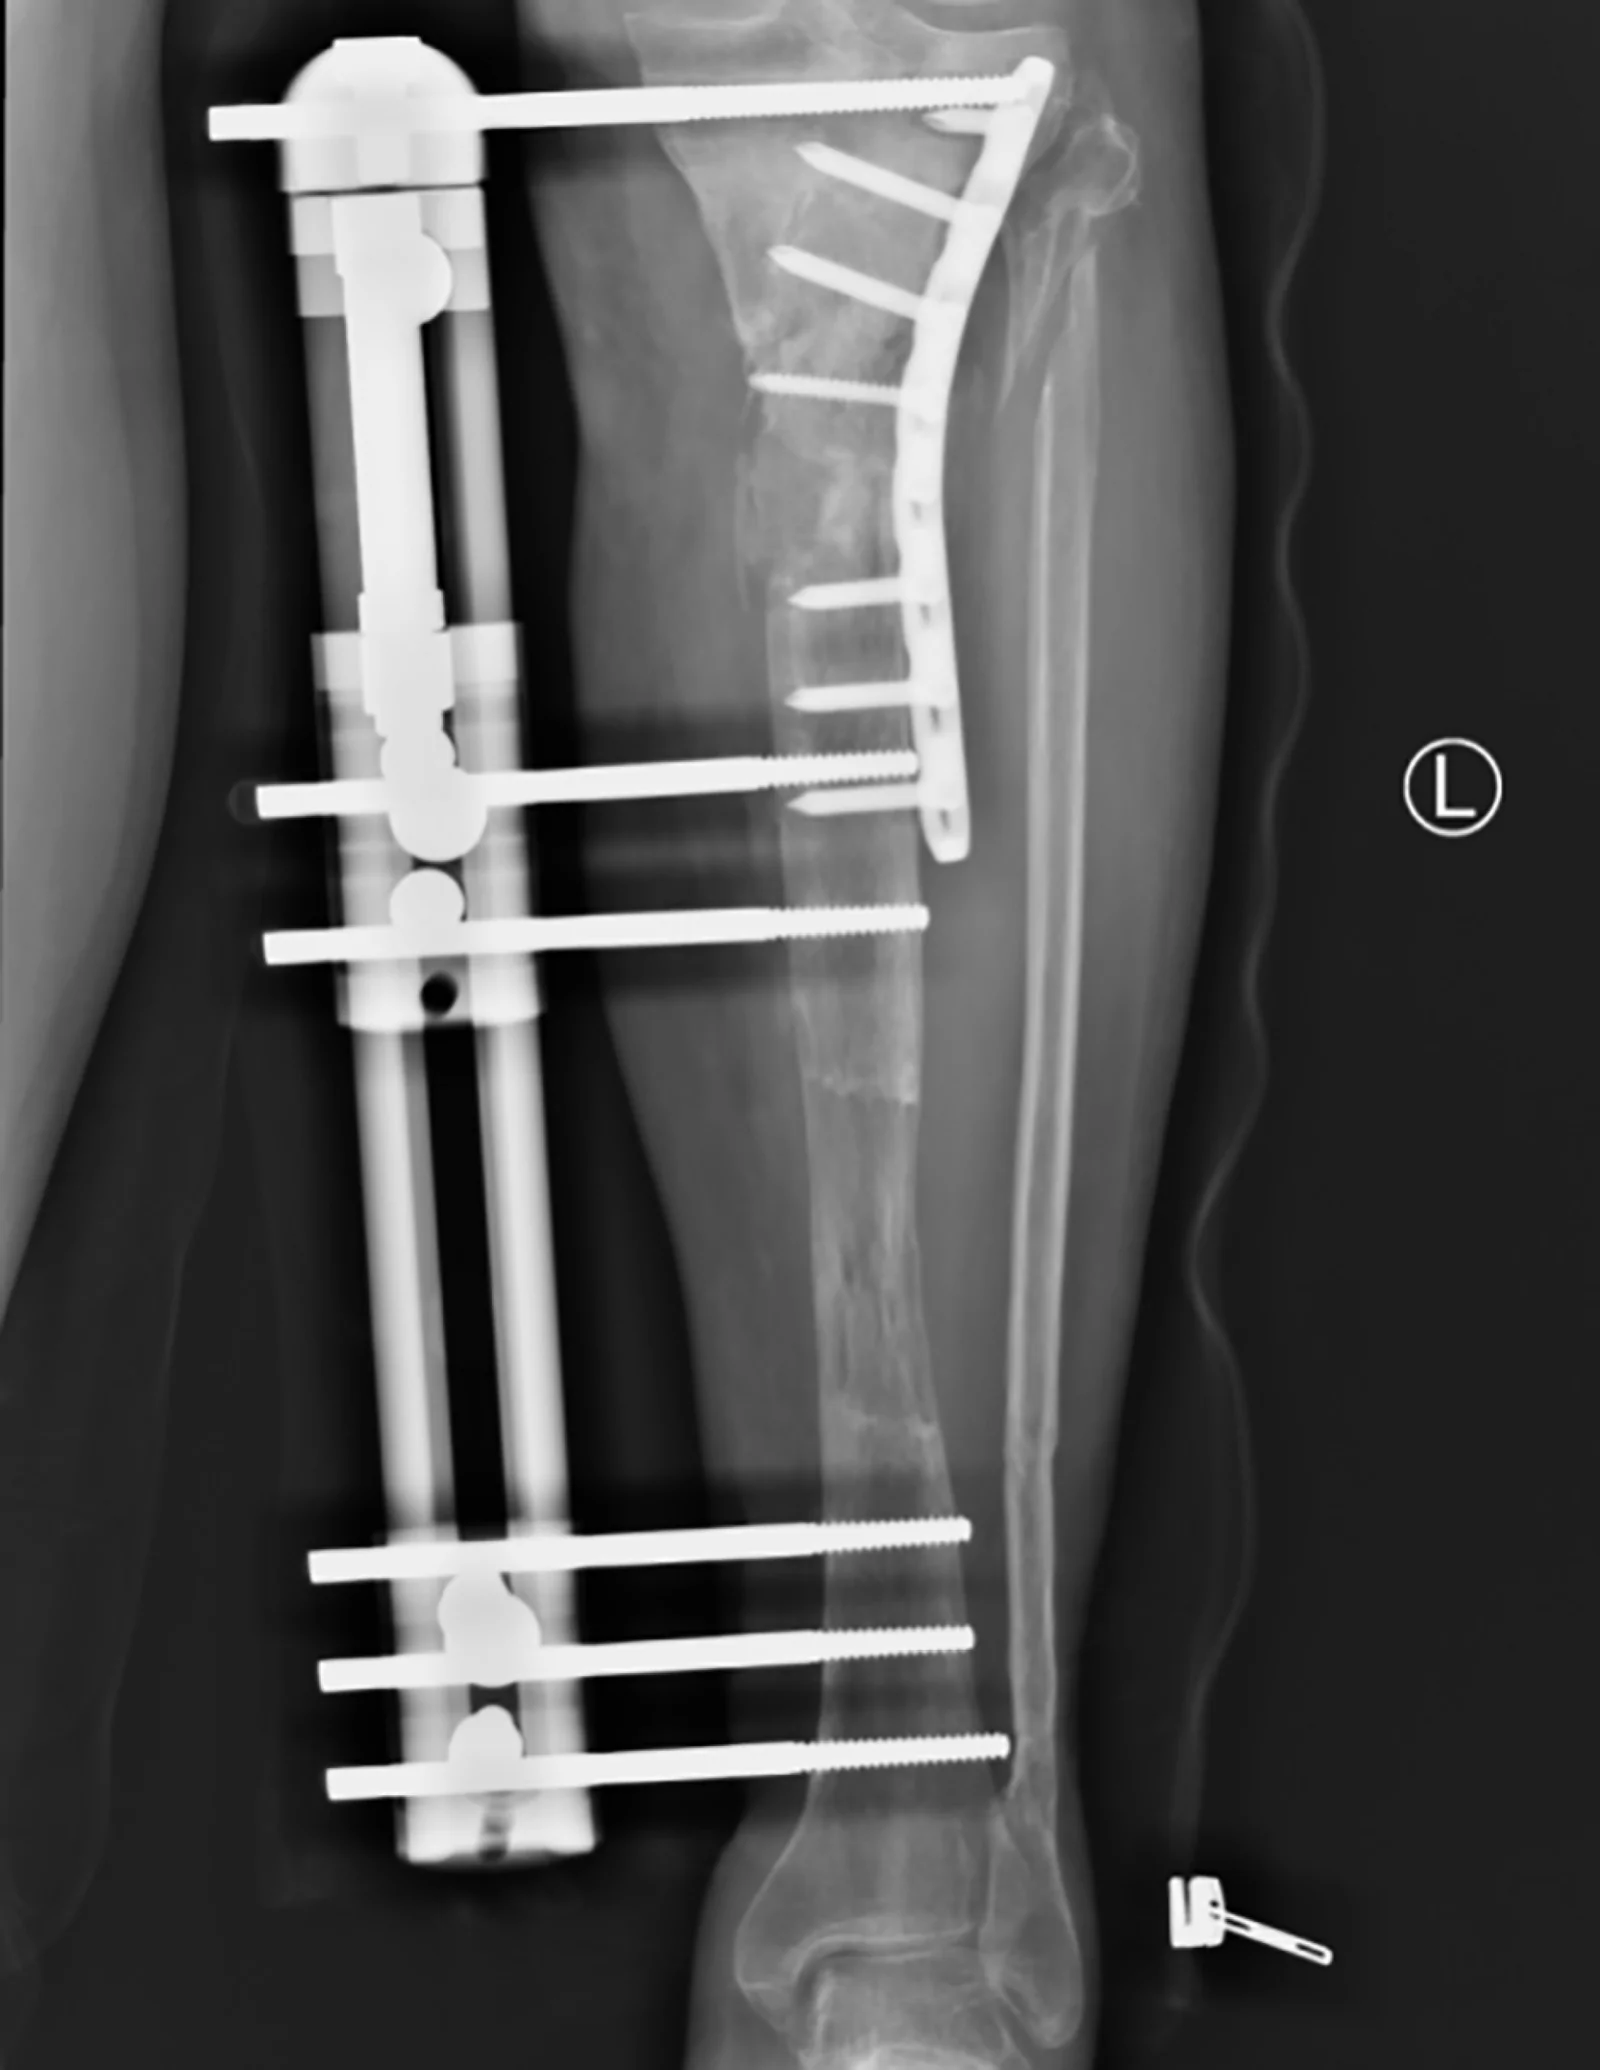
Follow-up radiograph at the latest outpatient visit (December 1, 2024). The fracture line at the docking site is progressively blurring, indicating ongoing consolidation. The regenerate bone in the distraction zone continues to mature with increased density, suggesting favorable bone healing.
